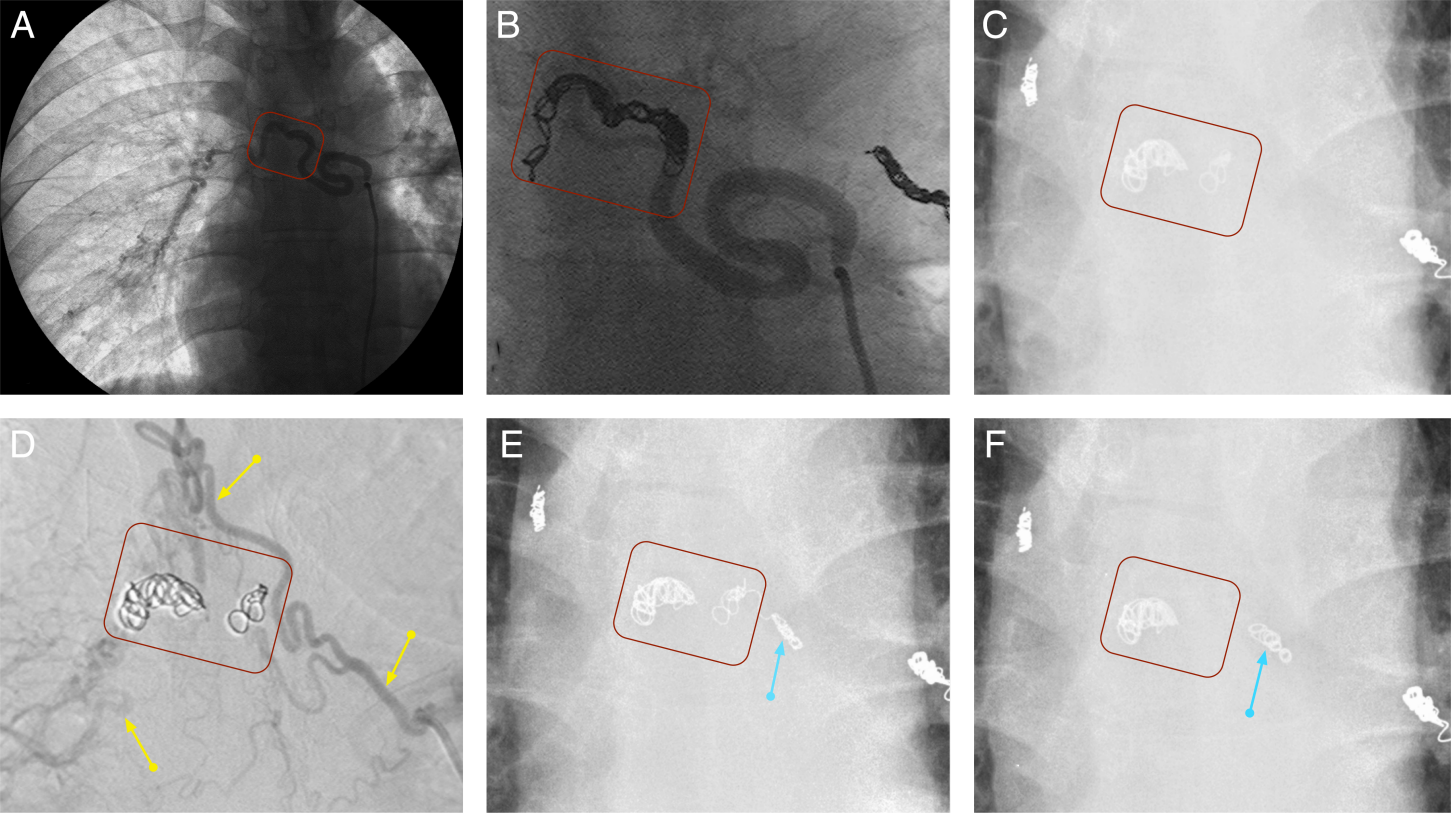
Case 1: Serial changes of the coils. (A) A right lower bronchial arteriography just before the embolization in the first super‐selective bronchial artery coil embolization (ssBACE) session 4.5 years before coughing up coil fragments. The red rectangle shows the position where the coils were going to be installed. (B) A cineangiography result of the first ssBACE of the right lower bronchial artery. The red rectangle shows the coils installed. (C) Chest radiography results after the patient coughed up coil fragments. The red rectangle shows the coils previously placed in the right lower bronchial artery. The depleted part in comparison to (B) was presumed to explain the coughed‐up coils. (D) A cineangiography result of the right upper bronchial artery (yellow arrow). We found that it was supplying collateral blood flow to both distal and proximal parts of the embolized area of the right lower bronchial artery. We suspected that the low‐pressure collateral blood flow was the cause of slight bleeding in the elevated area in the left main bronchus (see Fig. 2D, E). (E) The right upper bronchial artery was embolized. Blue arrow indicates the newly installed coils. (F) Approximately eight months later, the patient revisited our hospital after having coughed up more coil fragments. Chest radiography showed that the coils in the right lower bronchial artery were further reduced (red rectangle). Blue arrow indicates the coils installed in the second ssBACE. They appeared a little looser than (E); however, the coil quantity was presumed unchanged.
